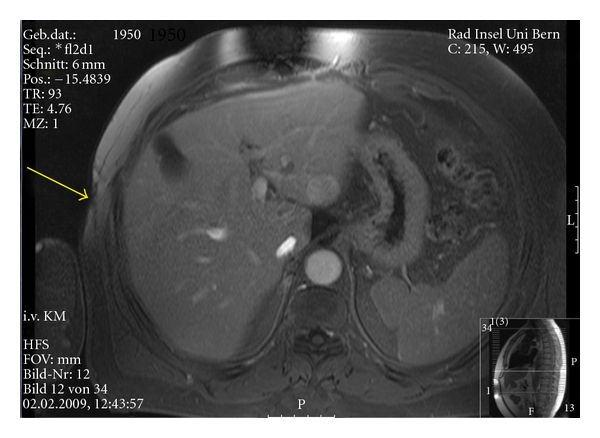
Magnetic resonance imaging (MRI) showing changes in the subcutaneous tissue extending to the ribs.
